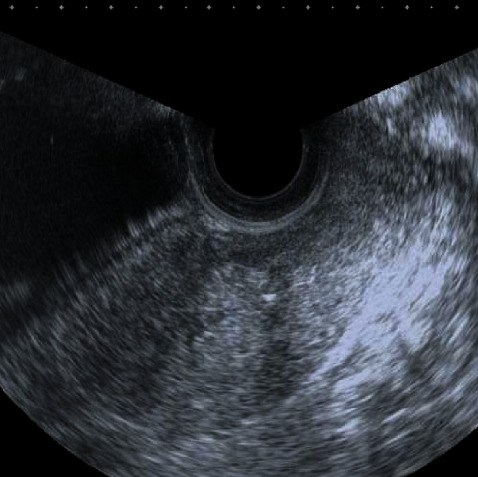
Ultrasound view of the uterus 1 month after childbirth. The longitudinal length of the uterus is 8.2 cm. No abnormal structures are visible in either the uterine body or the cervix.
